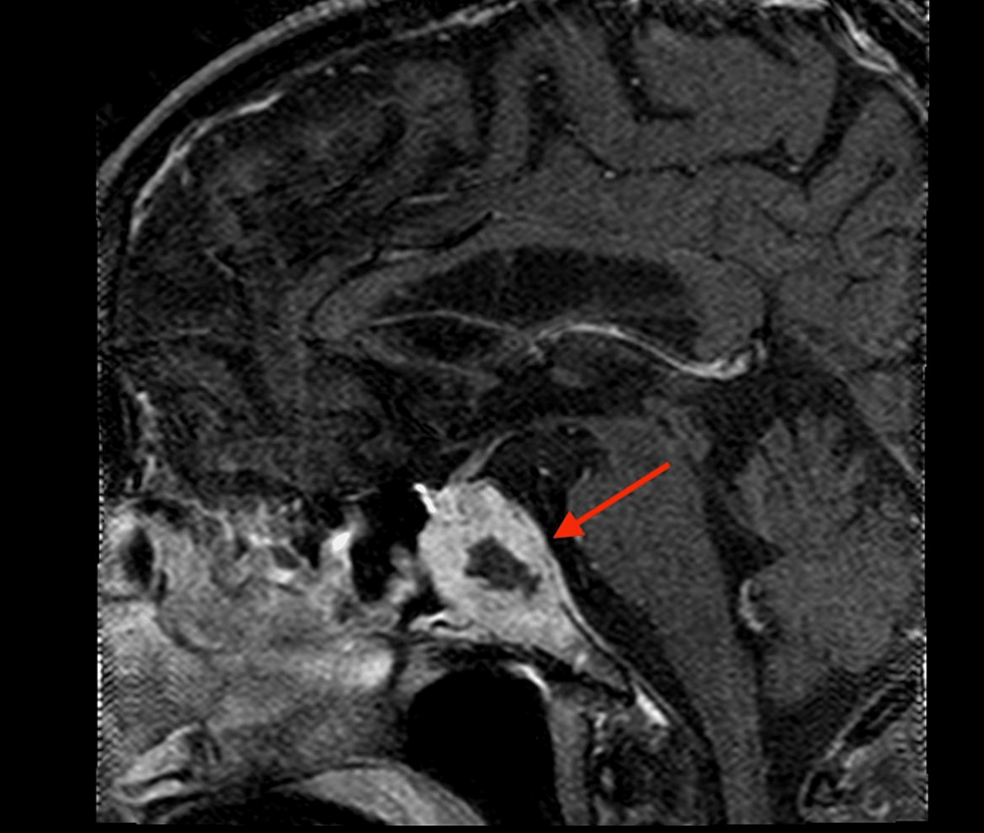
Sagittal MRI of the metastatic lesion abutting the optic chiasm (arrow).
